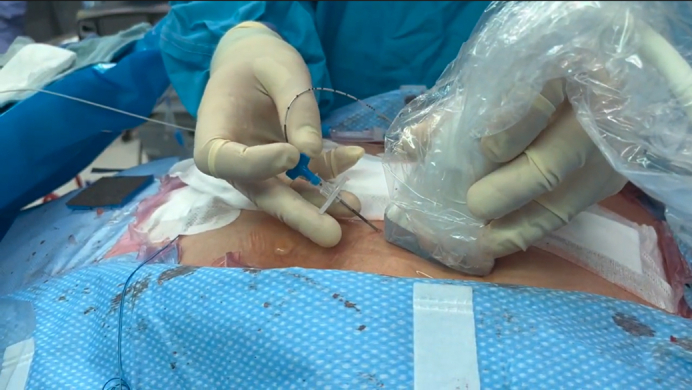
Insertion of the parasternal intercostal plane block catheter using ultrasound guidance.
